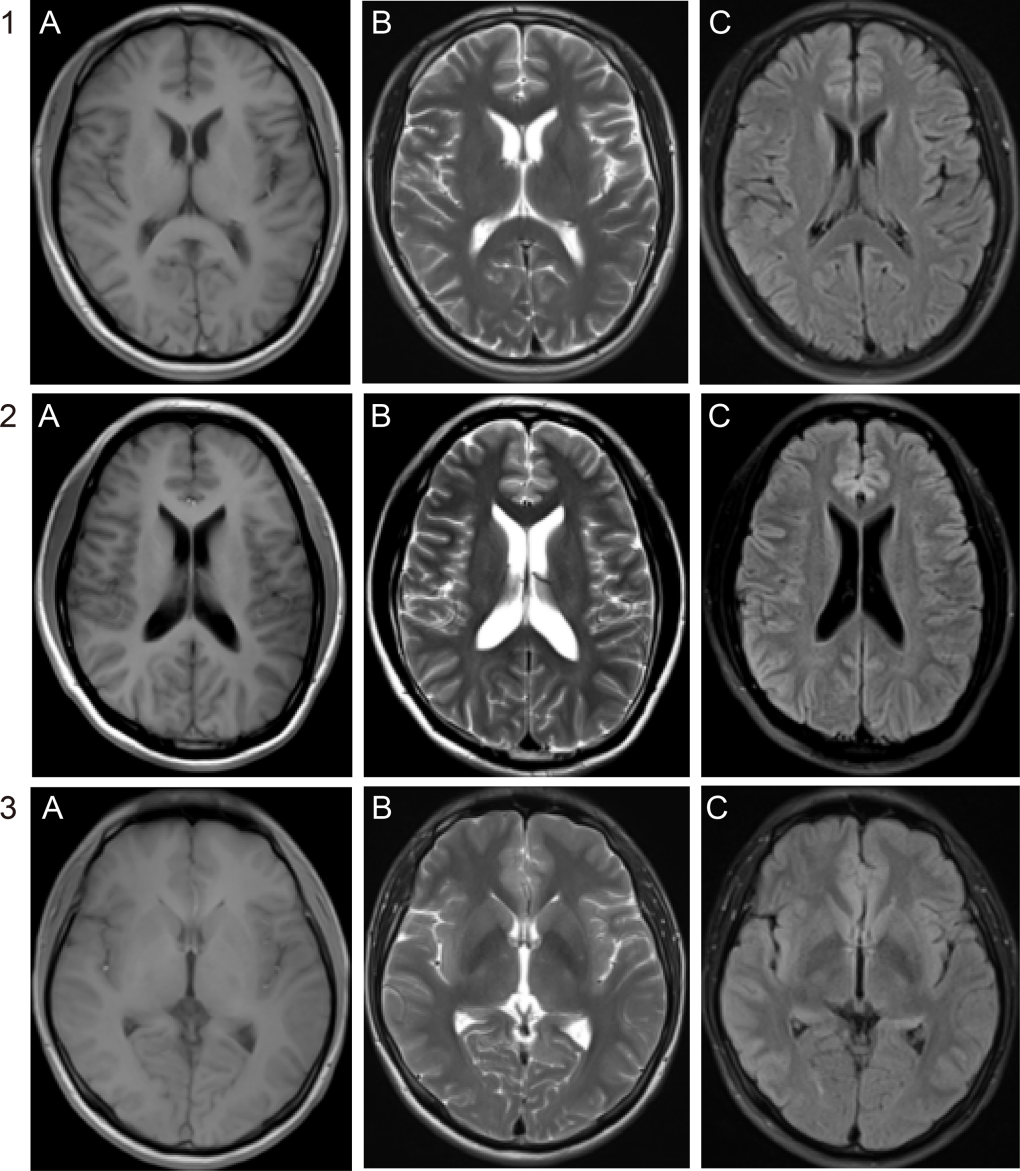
Brain MRI of the three patients. No abnormal signals were demonstrated on (A) T1WI, (B) T2WI, and (C) fluid-attenuated inversion recovery (FLAIR) of patients 1, 2, and 3.
